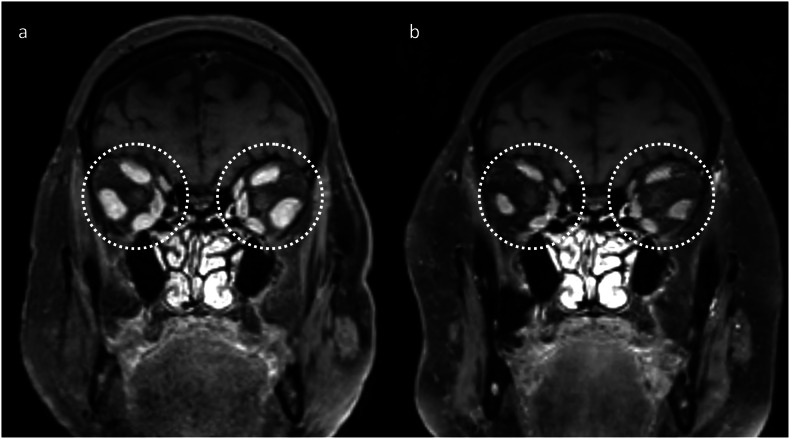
Post-contrast T1-weighted imaging in the coronal plane in a patient who had recently commenced pembrolizumab for a urothelial carcinoma. This demonstrates enlargement of the extraocular muscles (a, dotted circles), which resolved after treatment with corticosteroids (b), consistent with orbital myositis
